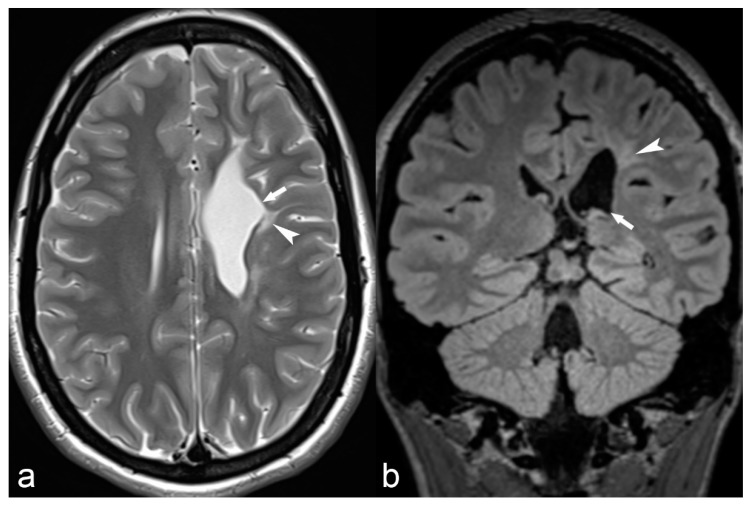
20-year-old female with epilepsy. Axial T2 weighted (a) and coronal FLAIR (b) images show irregularity of the contour of the left lateral ventricle with ex vacuo dilatation, hyperintensity and paucity of periventricular white matter (arrowhead) in the frontal region suggestive of sequalae of perinatal insult i.e., periventricular leukomalacia.
